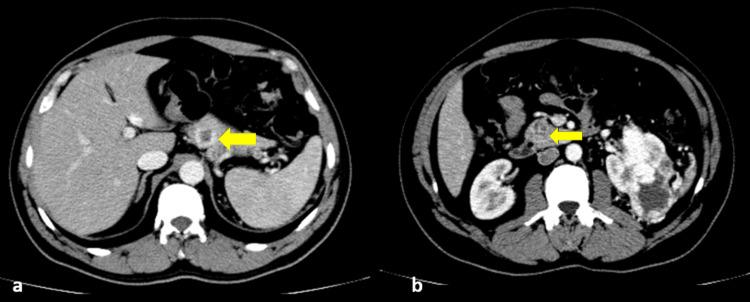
Contrast CT abdomen - axial (a and b) images demonstrate centrally enhancing lesions in the pancreas (yellow arrows) CT: computed tomography
